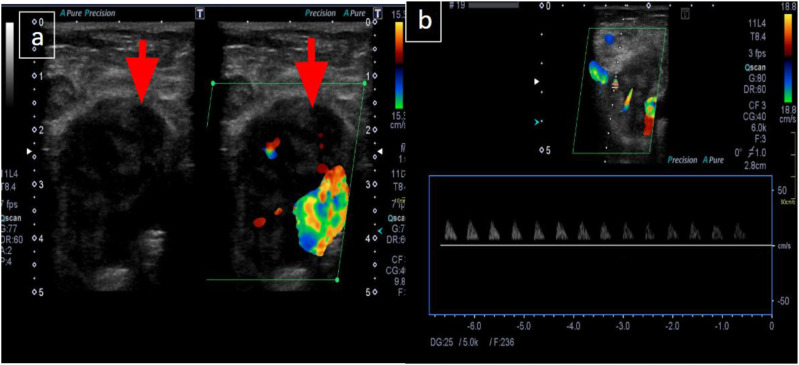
(A and B). Pelvic ultrasound demonstrating a vascular, lobulated hypoechoic lesion in the left inguinal region
